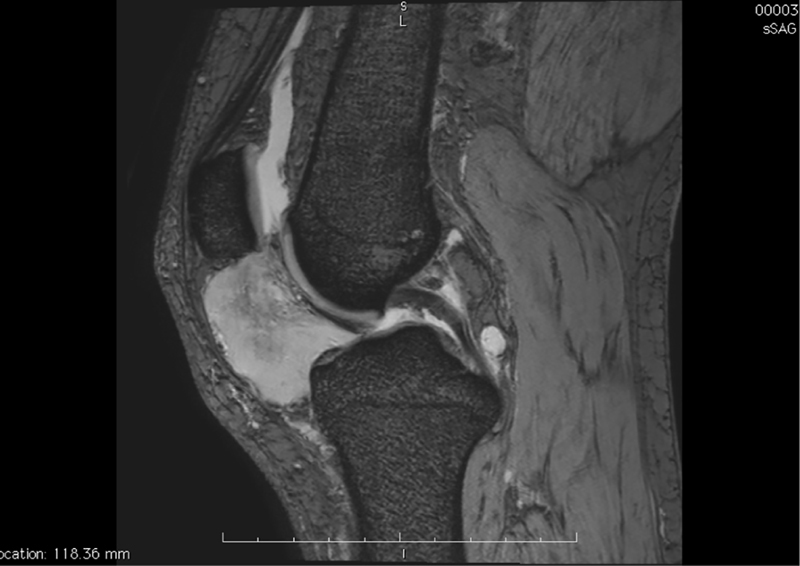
Sagittal T2-weighted MRI showing an infrapatellar schwannoma measuring 64 × 34 mm. MRI, magnetic resonance imaging.
